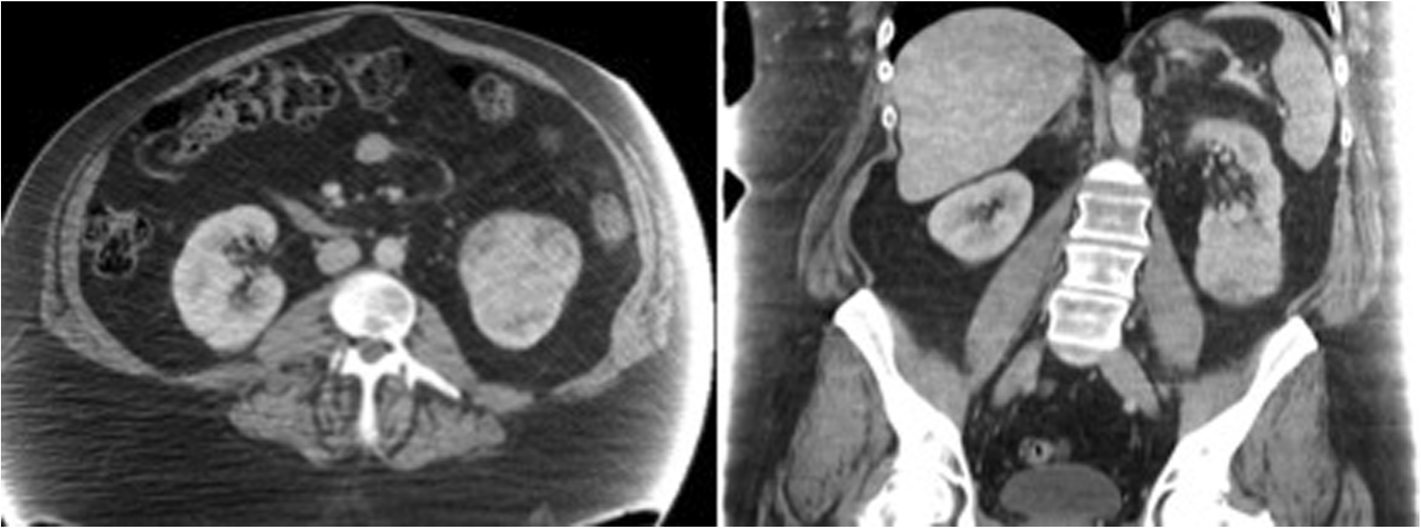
Abdominal computed tomography imaging of the patient shows a large exophytic heterogeneous mass in the lower pole of the left kidney
